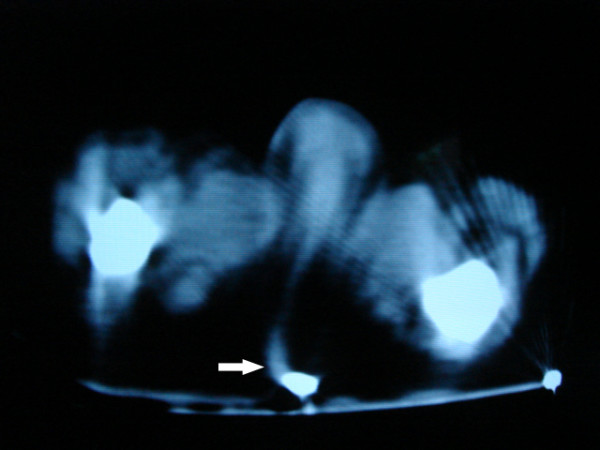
A computed tomogram verifying the central placement of the guide wire (arrow).
